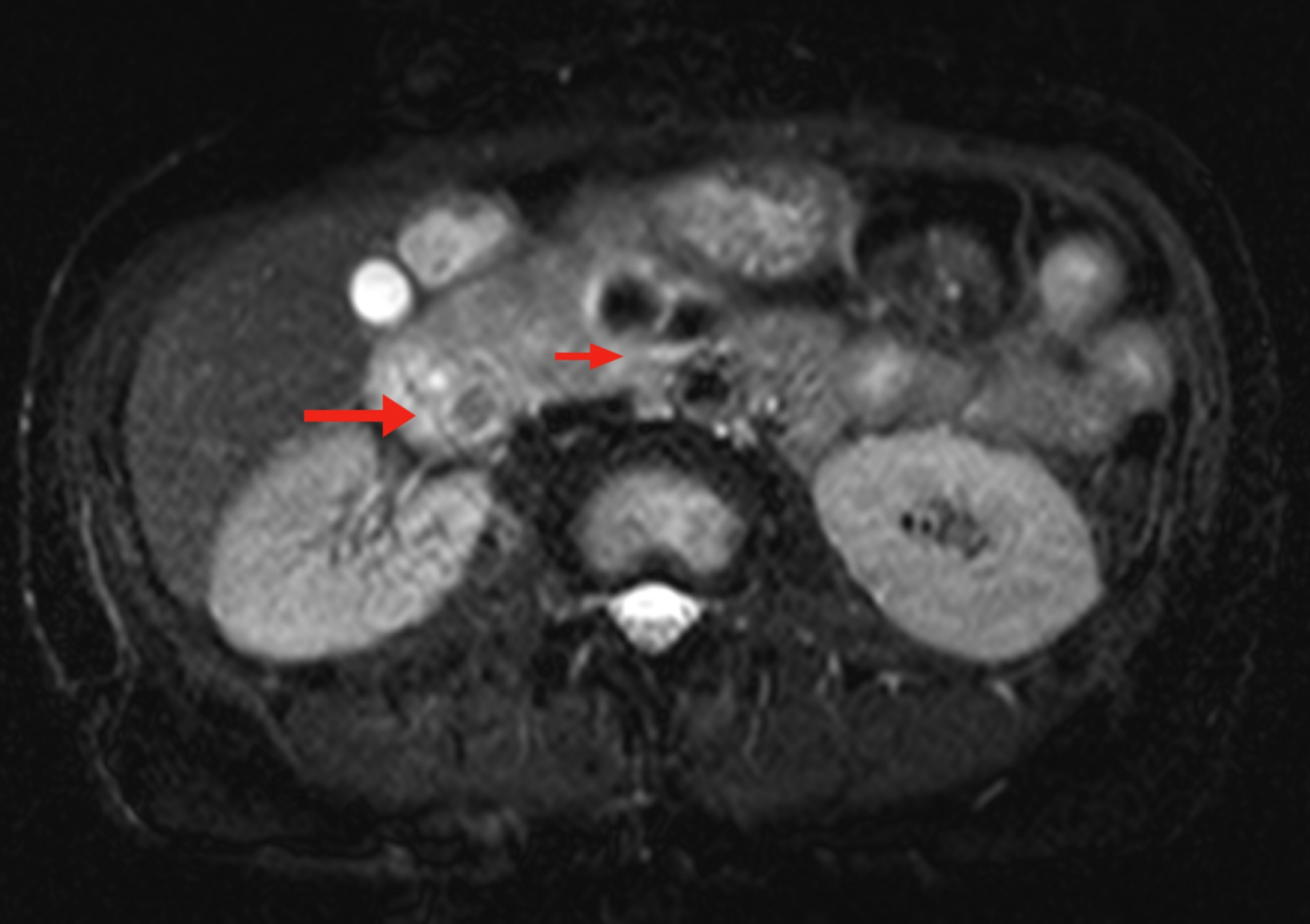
MRI of the pancreas on presentation Shown are the pancreatic head mass (large arrow) along with dilatation of the pancreatic duct (small arrow).
